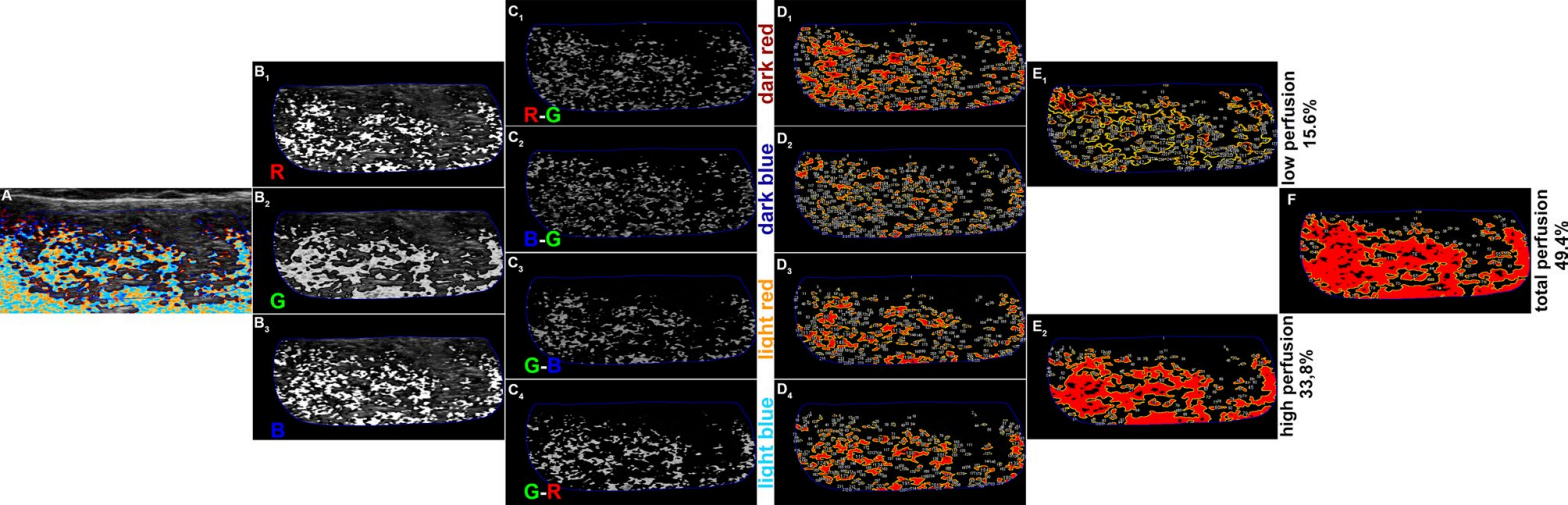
Analysis of color doppler myosonographs (A) performed by splitting of the channels (B), image subtraction steps (C) and signal selection of low and high velocity signals for both directions of blood perfusion (D). By creating maximum projections, direction-independent signals were achieved, but since the low velocity perfusion signal includes high velocity perfusion signal (see Supplemental Fig. S1D), the low velocity signal was corrected by subtraction of the high-velocity perfusion signal (E_1_). Subsequently, the corrected low velocity perfusion signal (E_1_) and the high velocity perfusion signal (E_2_) were merged in a maximum projection to gain the signal for the total blood perfusion of the muscle (F).
